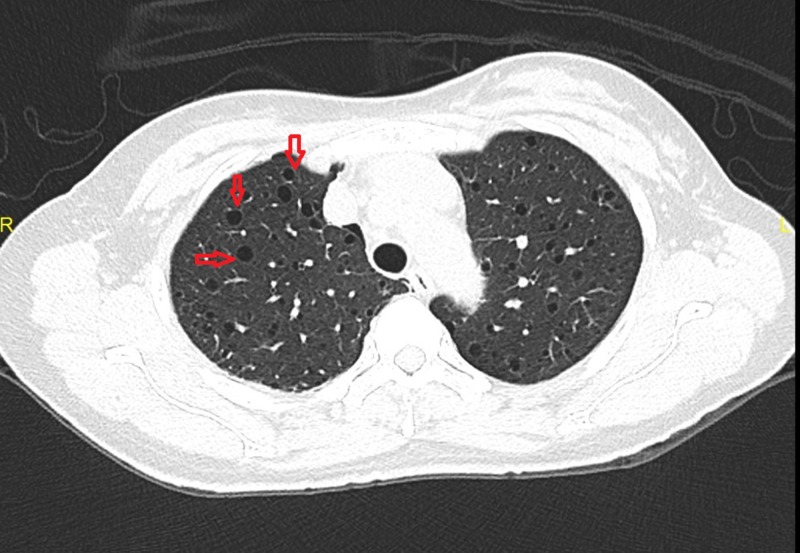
Sagittal section: Chest computed tomography scan showing multiple cysts
